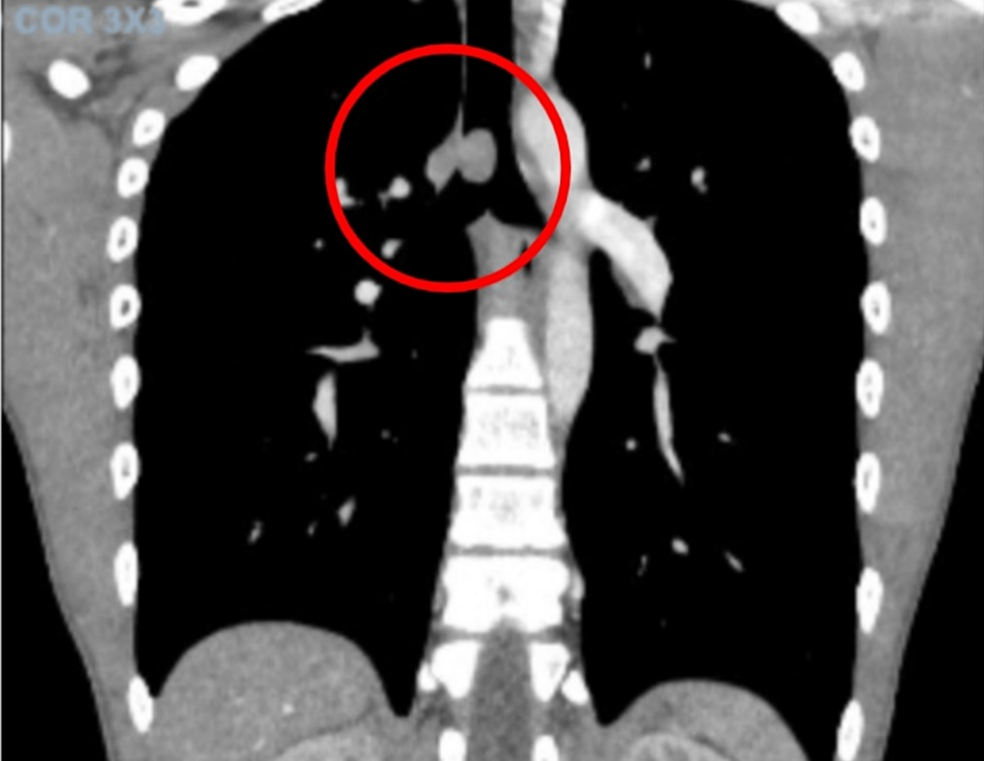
A computed tomography image with contrast depicting an endobronchial mass seen within the distal trachea (red circle) without atelectasis or infiltration. The mass involving the proximal part of the right bronchus was moderately obliterating the tracheal lumen and measured approximately 8 x 11 x 19 millimeters.
